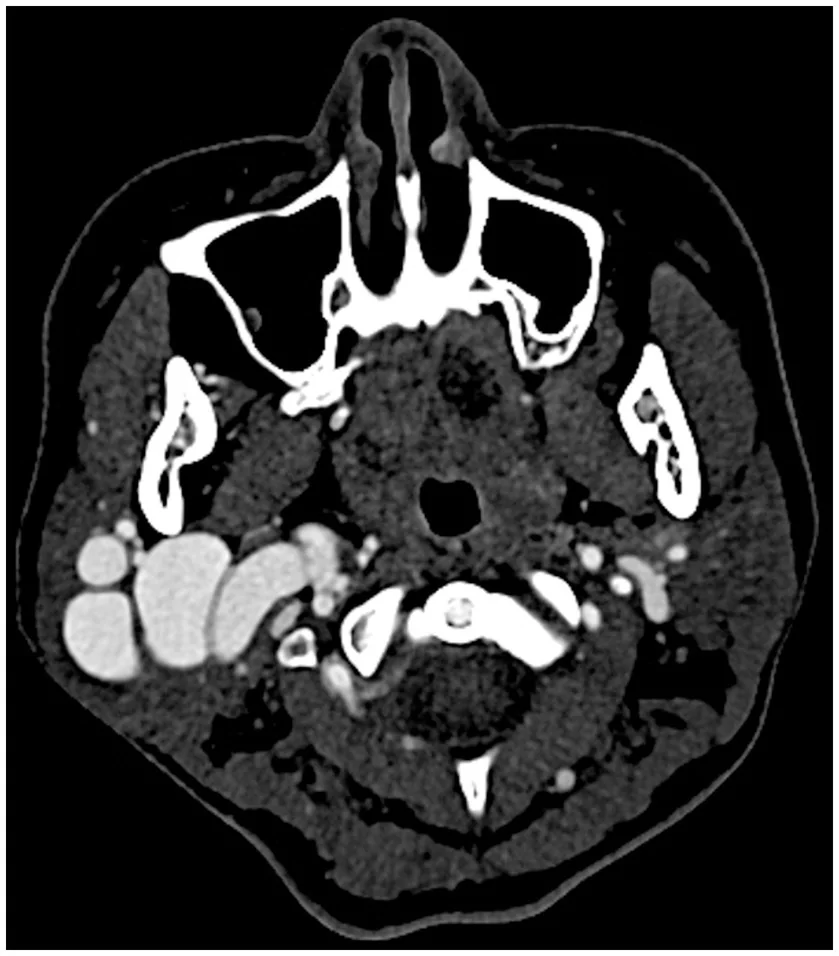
Contrast-enhanced computed tomography of a 35-year-old female with a parotid arteriovenous fistula (patient # 13). Ectatic vessels with intense enhancement were noted in the left parotid region.
